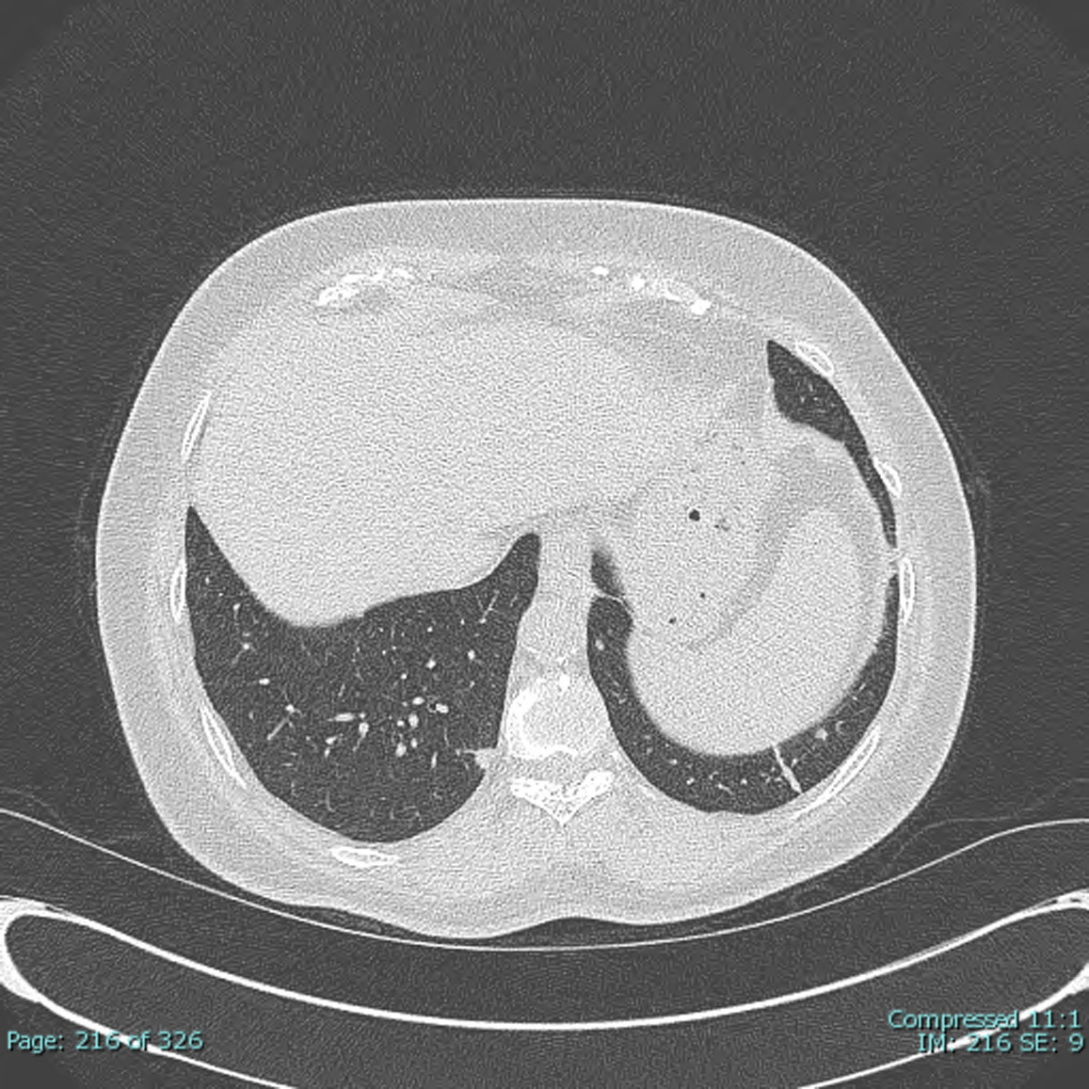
An axial noncontrast computed tomography (CT) slice at the lower chest in the lung window shows a new 6 mm juxta pleural nodule along the medial margin of the right lower lobe.
